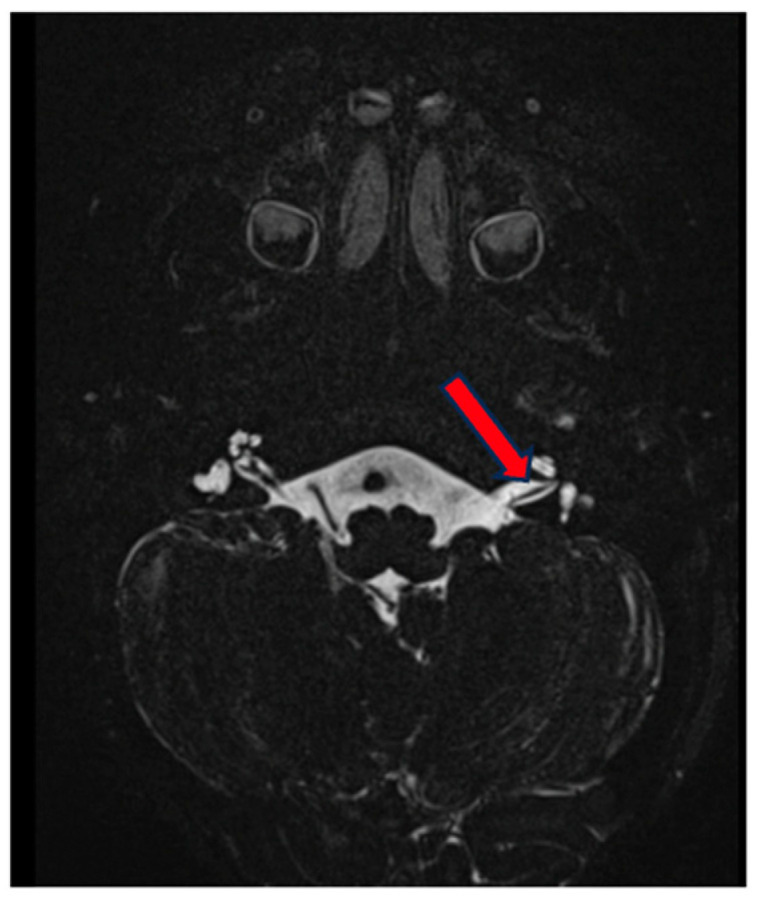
MRI of the inner ear for case 2, T2 tse3d weighted image, hypoplasia of the left cochlear nerve (red arrow).
